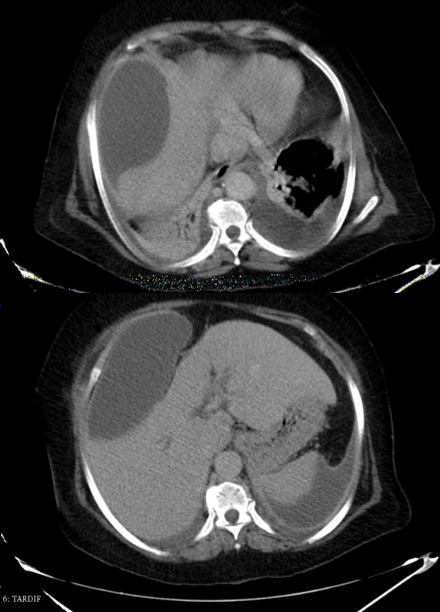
Abdominal CT demonstrating a large right hepatic subcapsular collection (black arrow)
